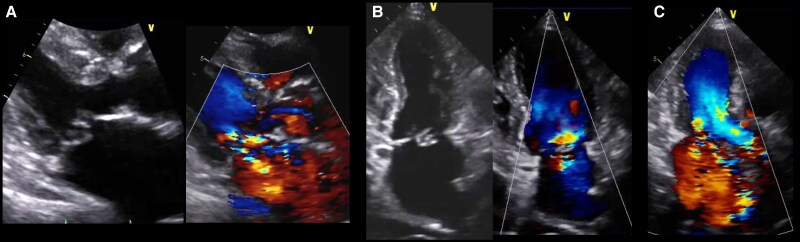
Severe mitral regurgitation with a posterior leaflet prolapse detected by transthoracic echocardiography on admission: (*A*) parasternal long axis view, (*B*) apical two-chamber view, and (*C*) apical three-chamber view.
